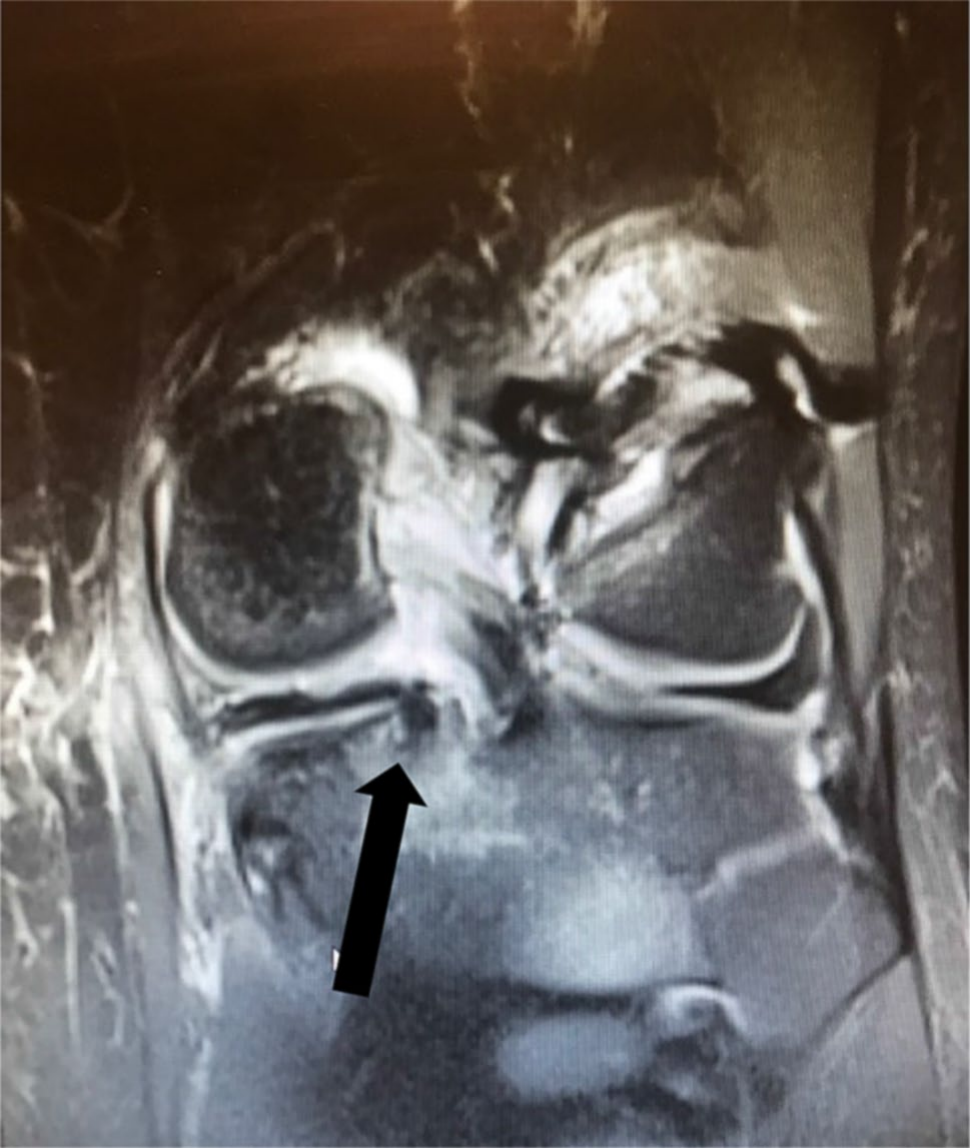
Frontal view of MRI T2-scan showing a medial meniscus transplant with the posterior root attachment (arrow)
